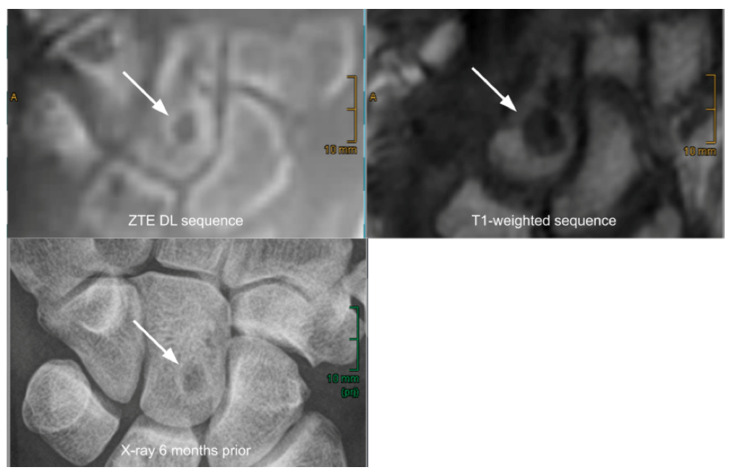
Forty-two-year-old male with swollen hands, in whom MRI was performed to assess the soft tissues, imaged with coronal ZTE DL (left) and T1-weighted (right) MRI, with a corresponding radiograph obtained 6 months earlier (bottom). An intraosseous lesion in the Os capitatum, most probably in keeping with fibro-osseous rests, is more clearly visualized on ZTE DL, with an overall CT-like appearance, than on T1-weighted imaging.
